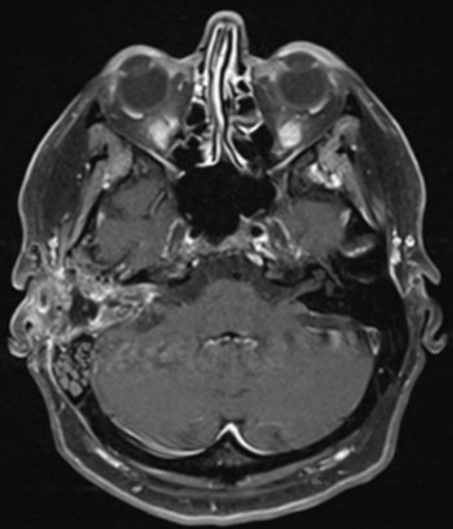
MRI 3 months after surgery, axial, T1 FS + CM. No recurrence after translabyrinthine resection 3 months after surgery.
